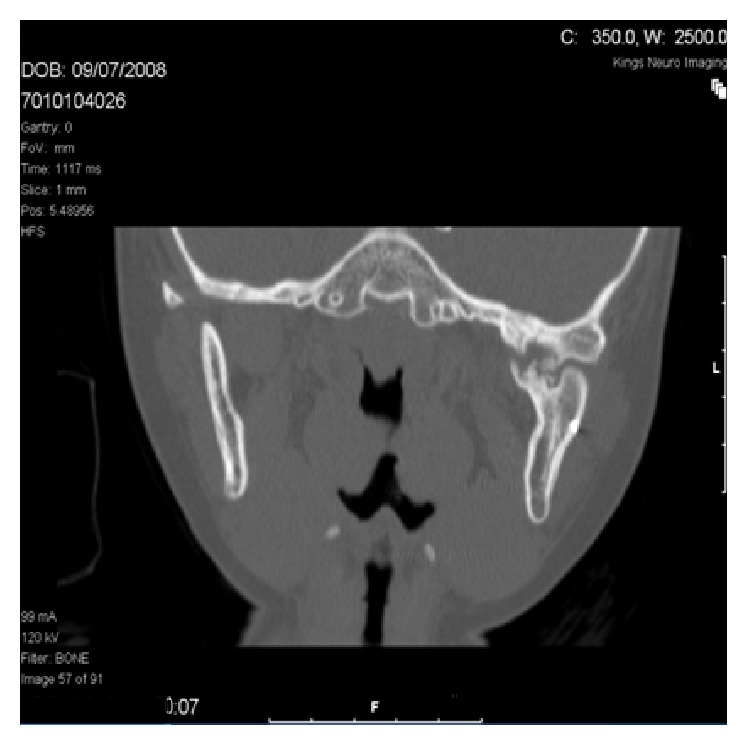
Coronal view of CT scan.
